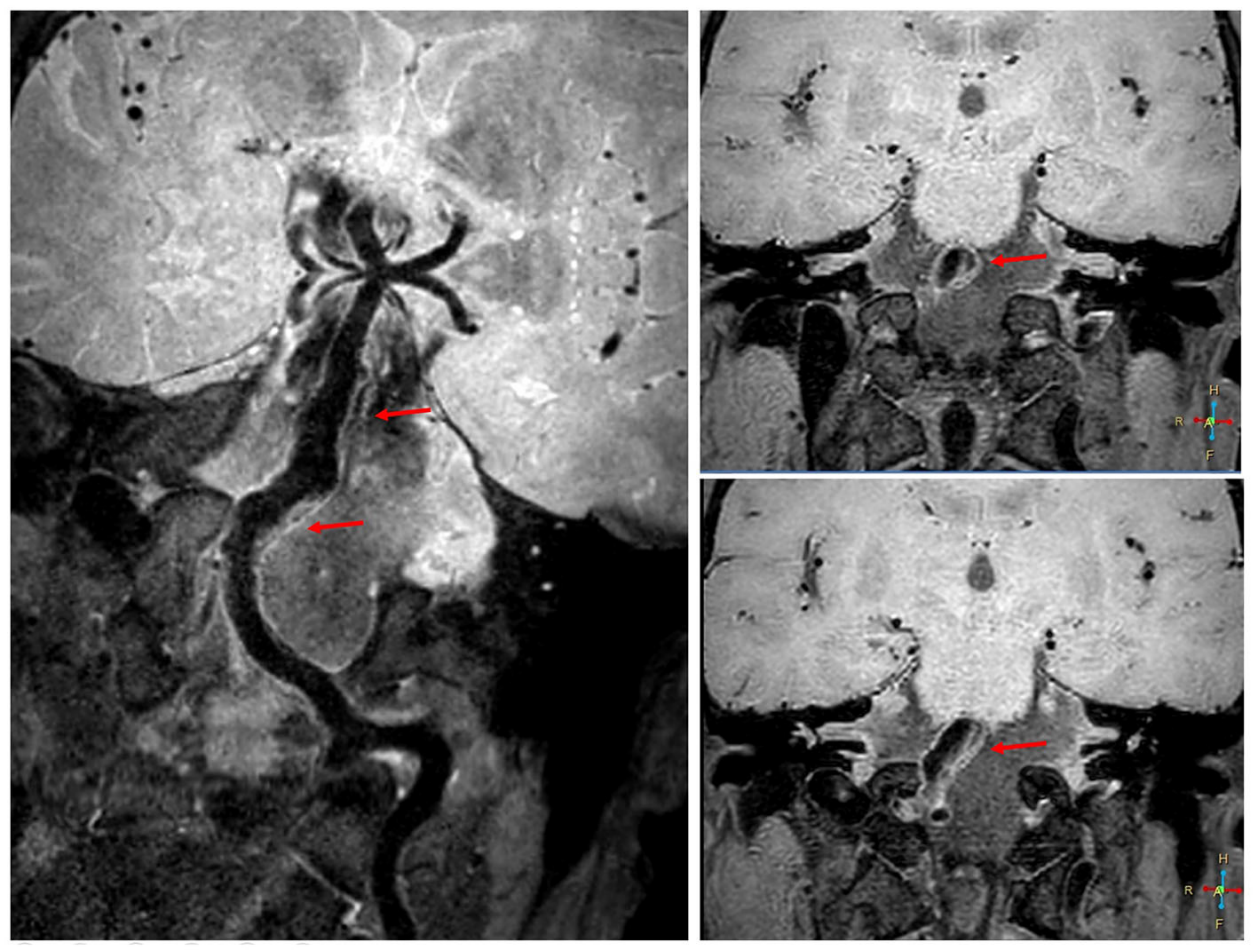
High resolution magnetic resonance imaging before the operation. High resolution magnetic resonance imaging reveals a vertebrobasilar dissecting aneurysm (red arrow).
